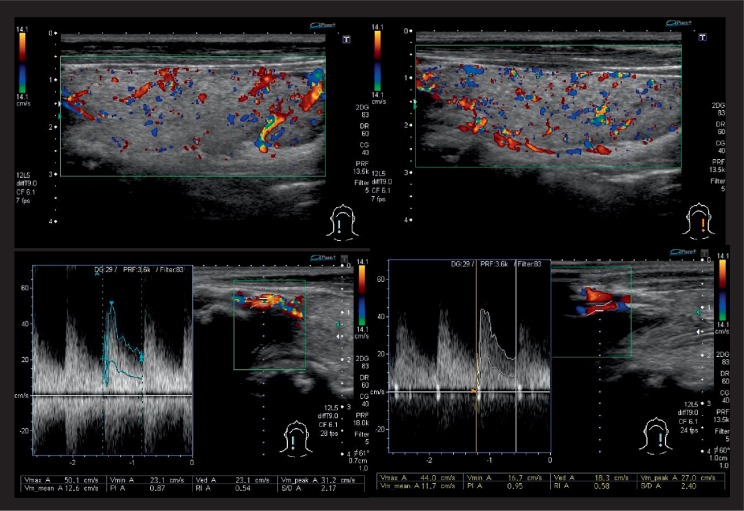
Thyroid ultrasonography performed the day after iodine treatment. Upper: Left is the intrathyroidal blood flow signal of the right lobe, Right is that of the left lobe. Lower: Left is right superior thyroid artery flow velocity, Vmax is 50.1 cm/s, Right is left superior thyroid artery flow velocity, Vmax is 44.0 cm/s.
